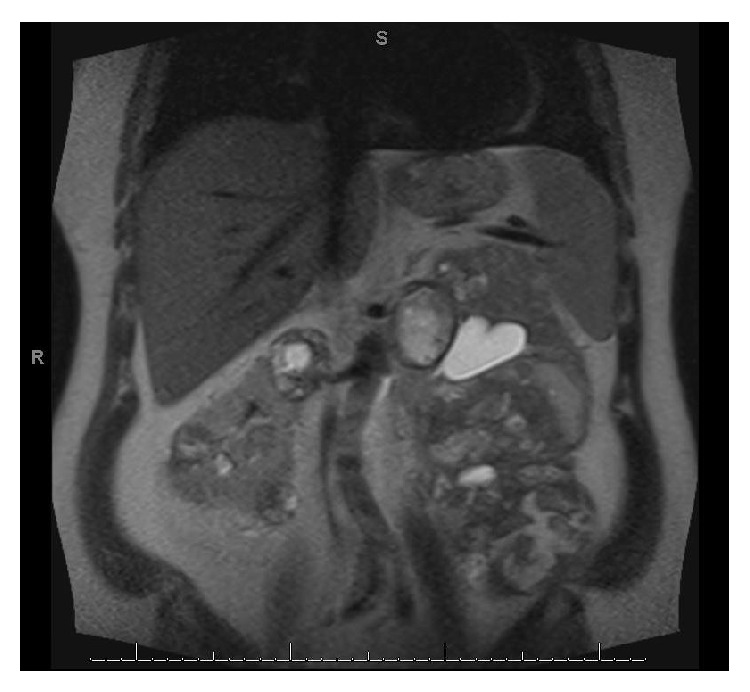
Preoperative abdominal MRI.
